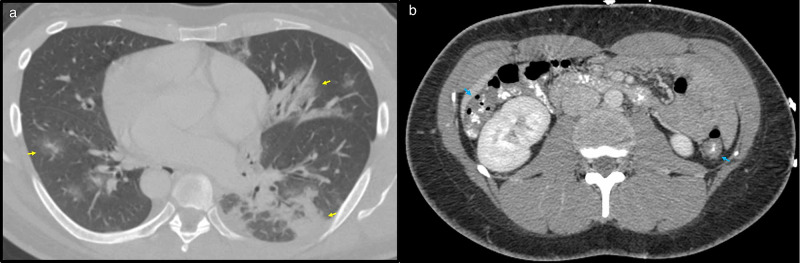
Axial image from a contrast-enhanced CT of the abdomen and pelvis (a) The image shows ground-glass opacities (yellow arrows) bilaterally at the lung bases and patchy consolidations (red arrows) in the left lower lobe. (b) In the upper abdomen, there is mild circumferential wall thickening of the transverse and descending colon (blue arrows) CT: computed tomography
